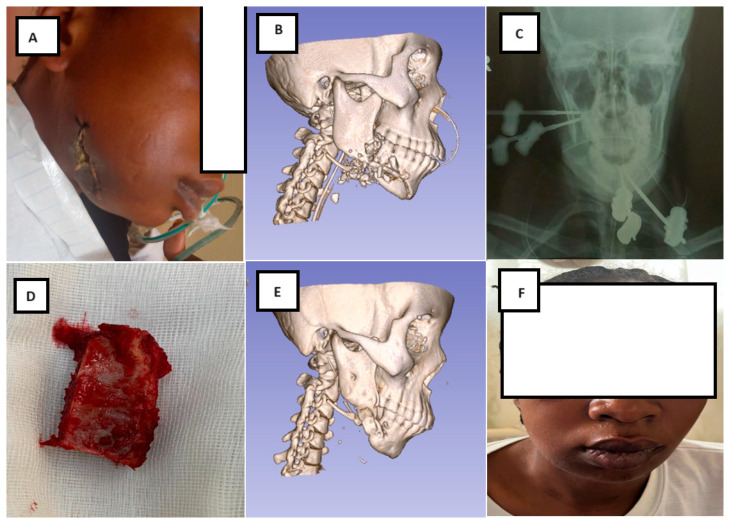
(A–C) A 36-year-old female patient with a high-velocity gunshot injury to the mandible, presenting with an extensive comminuted fracture and clinical evidence of wound infection at admission, treated with external fixation. (C) Postoperative radiograph showing pin placement, adequate fracture reduction, and a secondary bone defect following serial surgical debridement. (D) Corticocancellous iliac crest bone graft prepared for mandibular reconstruction. (E) Computed tomography (CT) scan obtained 6 months postoperatively demonstrating fracture healing and restoration of mandibular continuity. (F) Final clinical appearance at 6-month follow-up.
